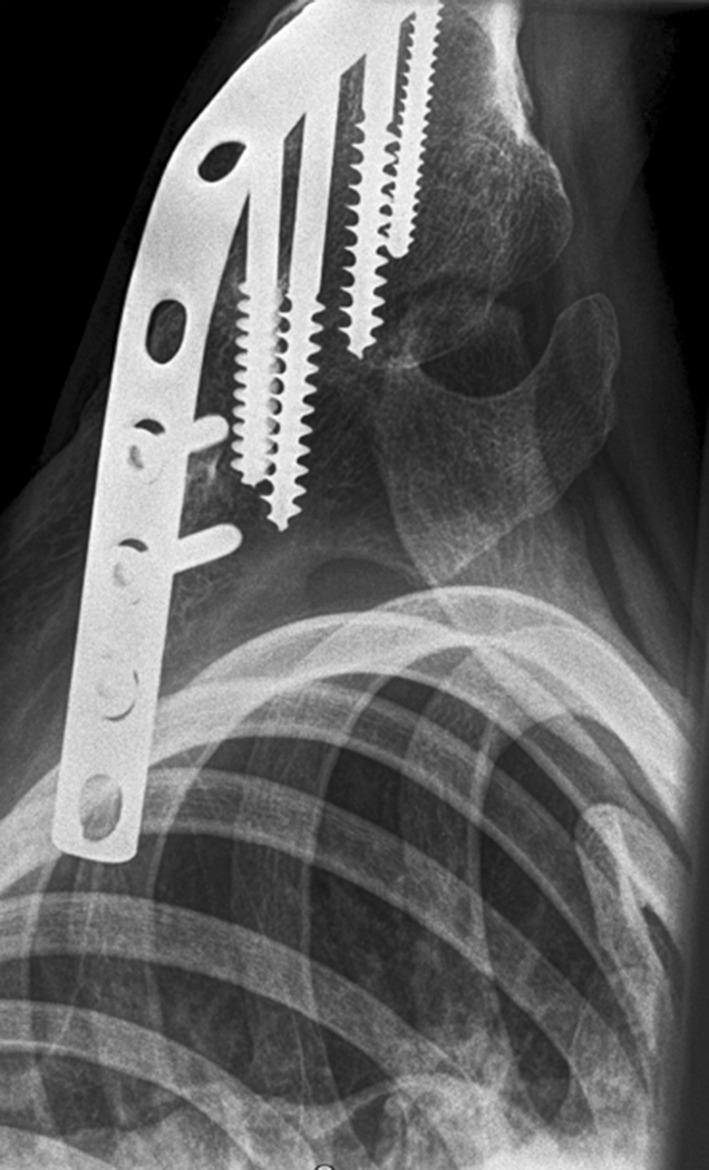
X-ray in axial view of the left shoulder of patient 12, 15.5-years after shoulder arthrodesis
